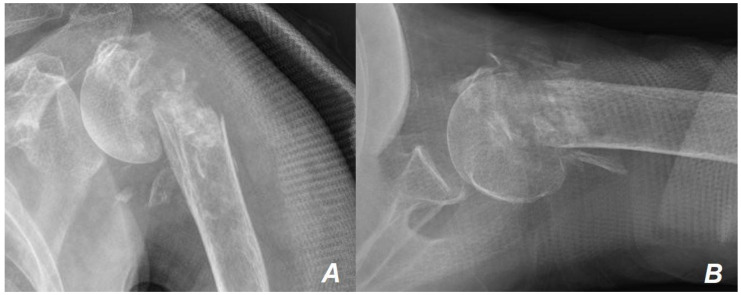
Initial plain radiographs. At the time of the visit, the initial plain radiographs revealed both medial and lateral cortical comminution of the proximal humeral metaphyseal area in the anterior-posterior (A) and trans-axillary (B) views of the X-ray images.
